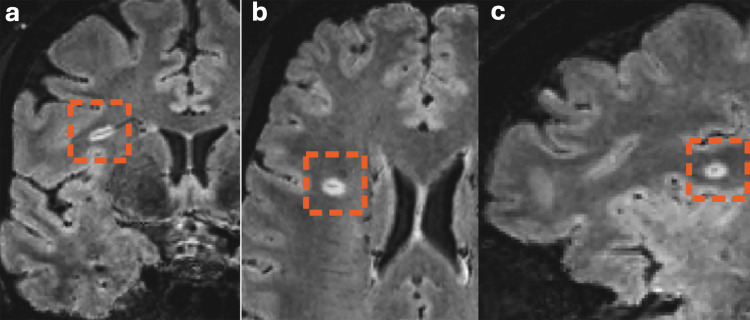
A zoomed-in (a) coronal, (b) axial, and (c) sagittal post-contrast T2 FLAIR* at 3T revealed CVS within the WM lesion indicated by the orange dotted rectangle. Reproduced from Rocca MA et al [3] under the terms of the Creative Commons Attribution License (CC BY 4.0).
